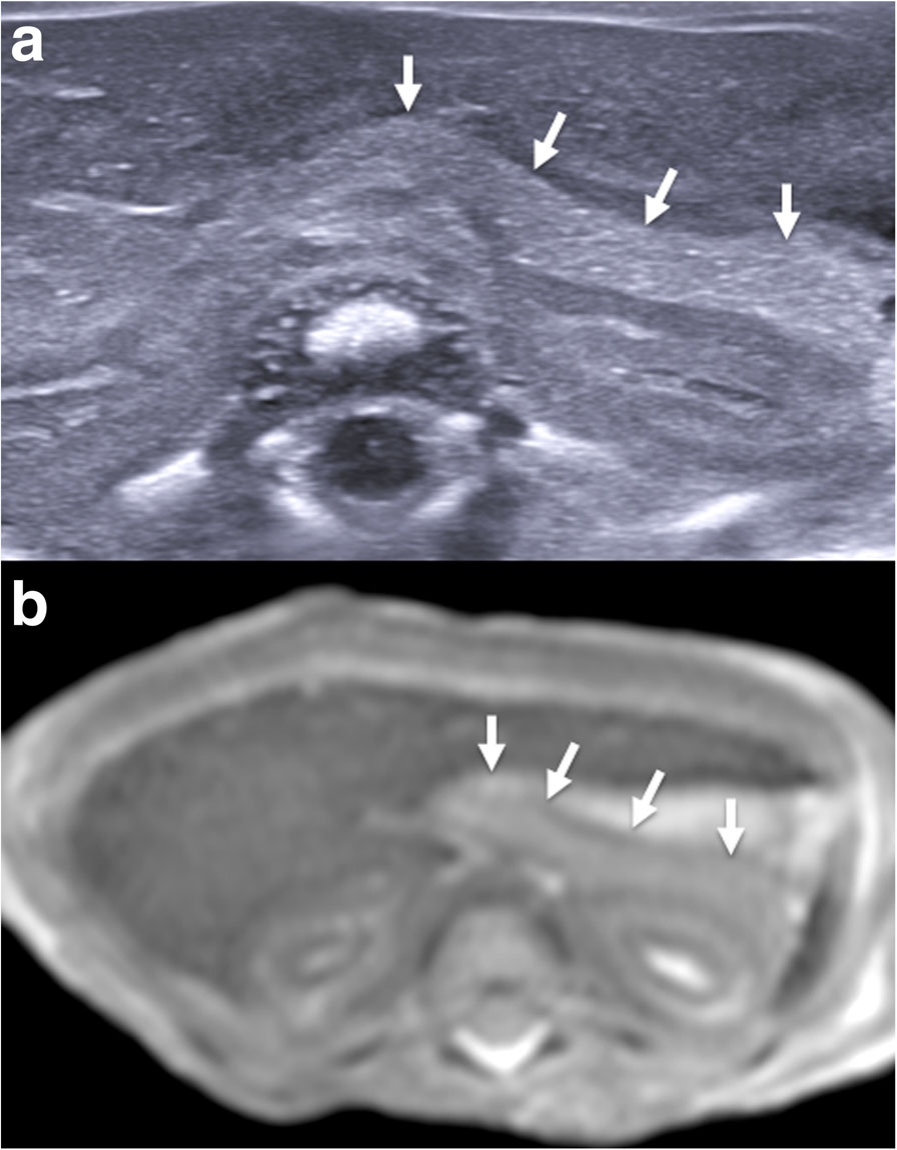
Normal appearances of the pancreas (white arrows) on transverse postmortem ultrasound (a) in a 20-week gestational-aged foetus, 4 days after death with corresponding postmortem T2-weighted MRI at 1.5 T (b) obtained 12 days after death
